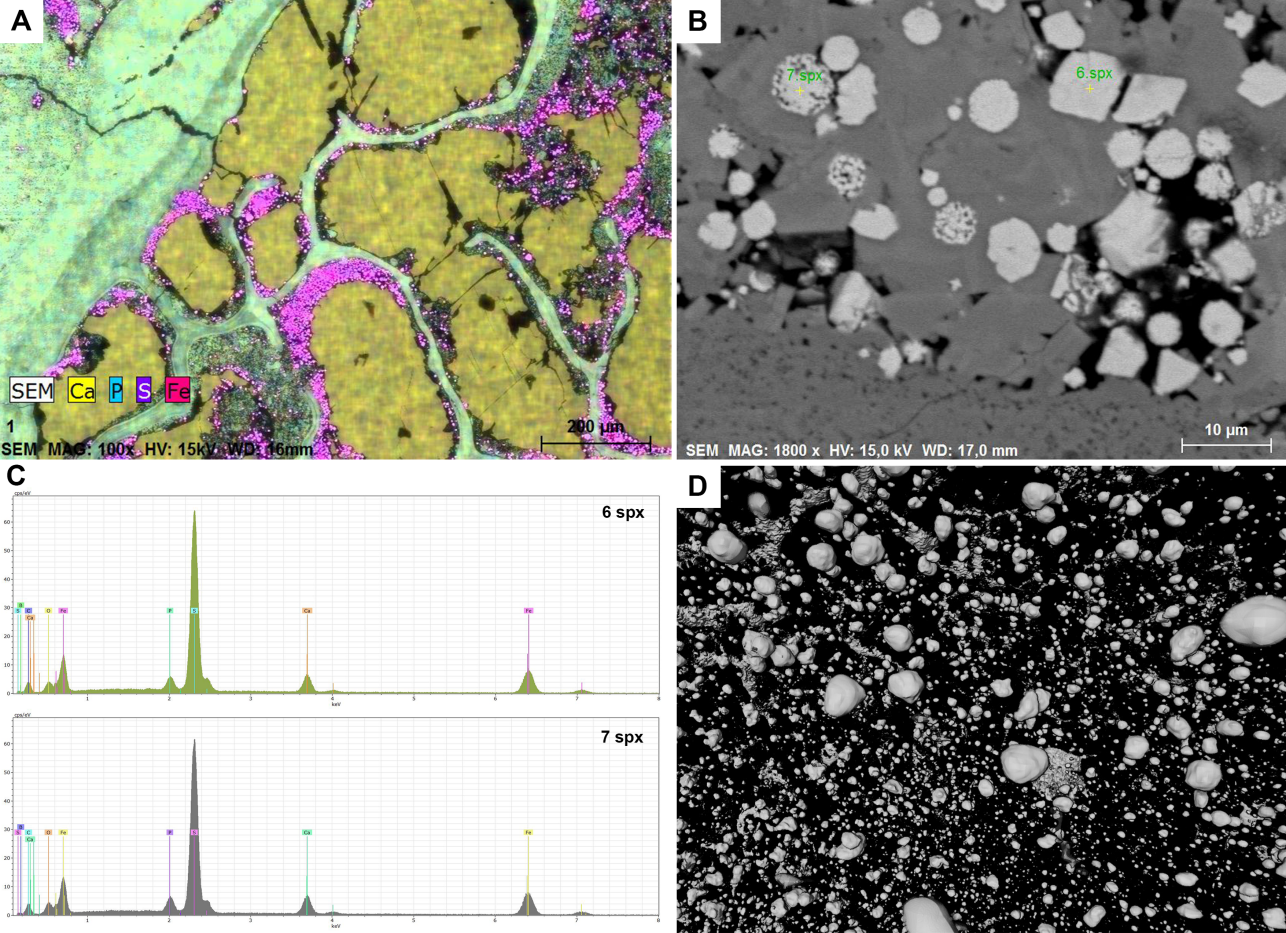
Chemical results. (A) SEM, Elemental map of PMO 250.004. The matrix and the bone consist of calcium phosphate. Pores inside bone cross section are filled with calcium carbonate. Pyrite grows in the outline of inclusion infill. (B) SEM picture including euhedral and framboid pyrite, PMO 250.004. (C) EDS results of measured points seen in picture B, PMO 250.004. (D) Pyrite seen in CT-reconstruction.
